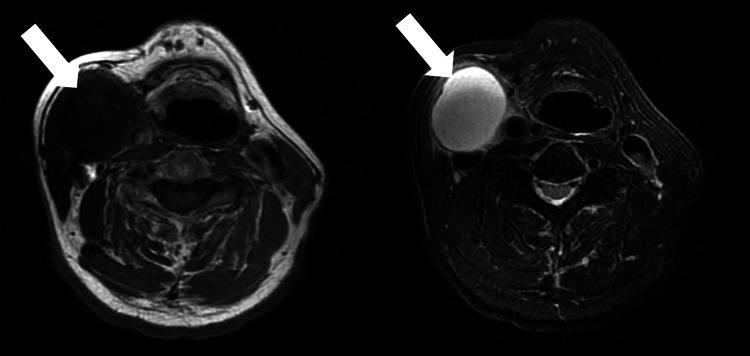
T1-weighted MRI (left) and T2-weighted MRI (right) A T1-hypointense/T2-hyperintense lesion (white arrow) was observed.
